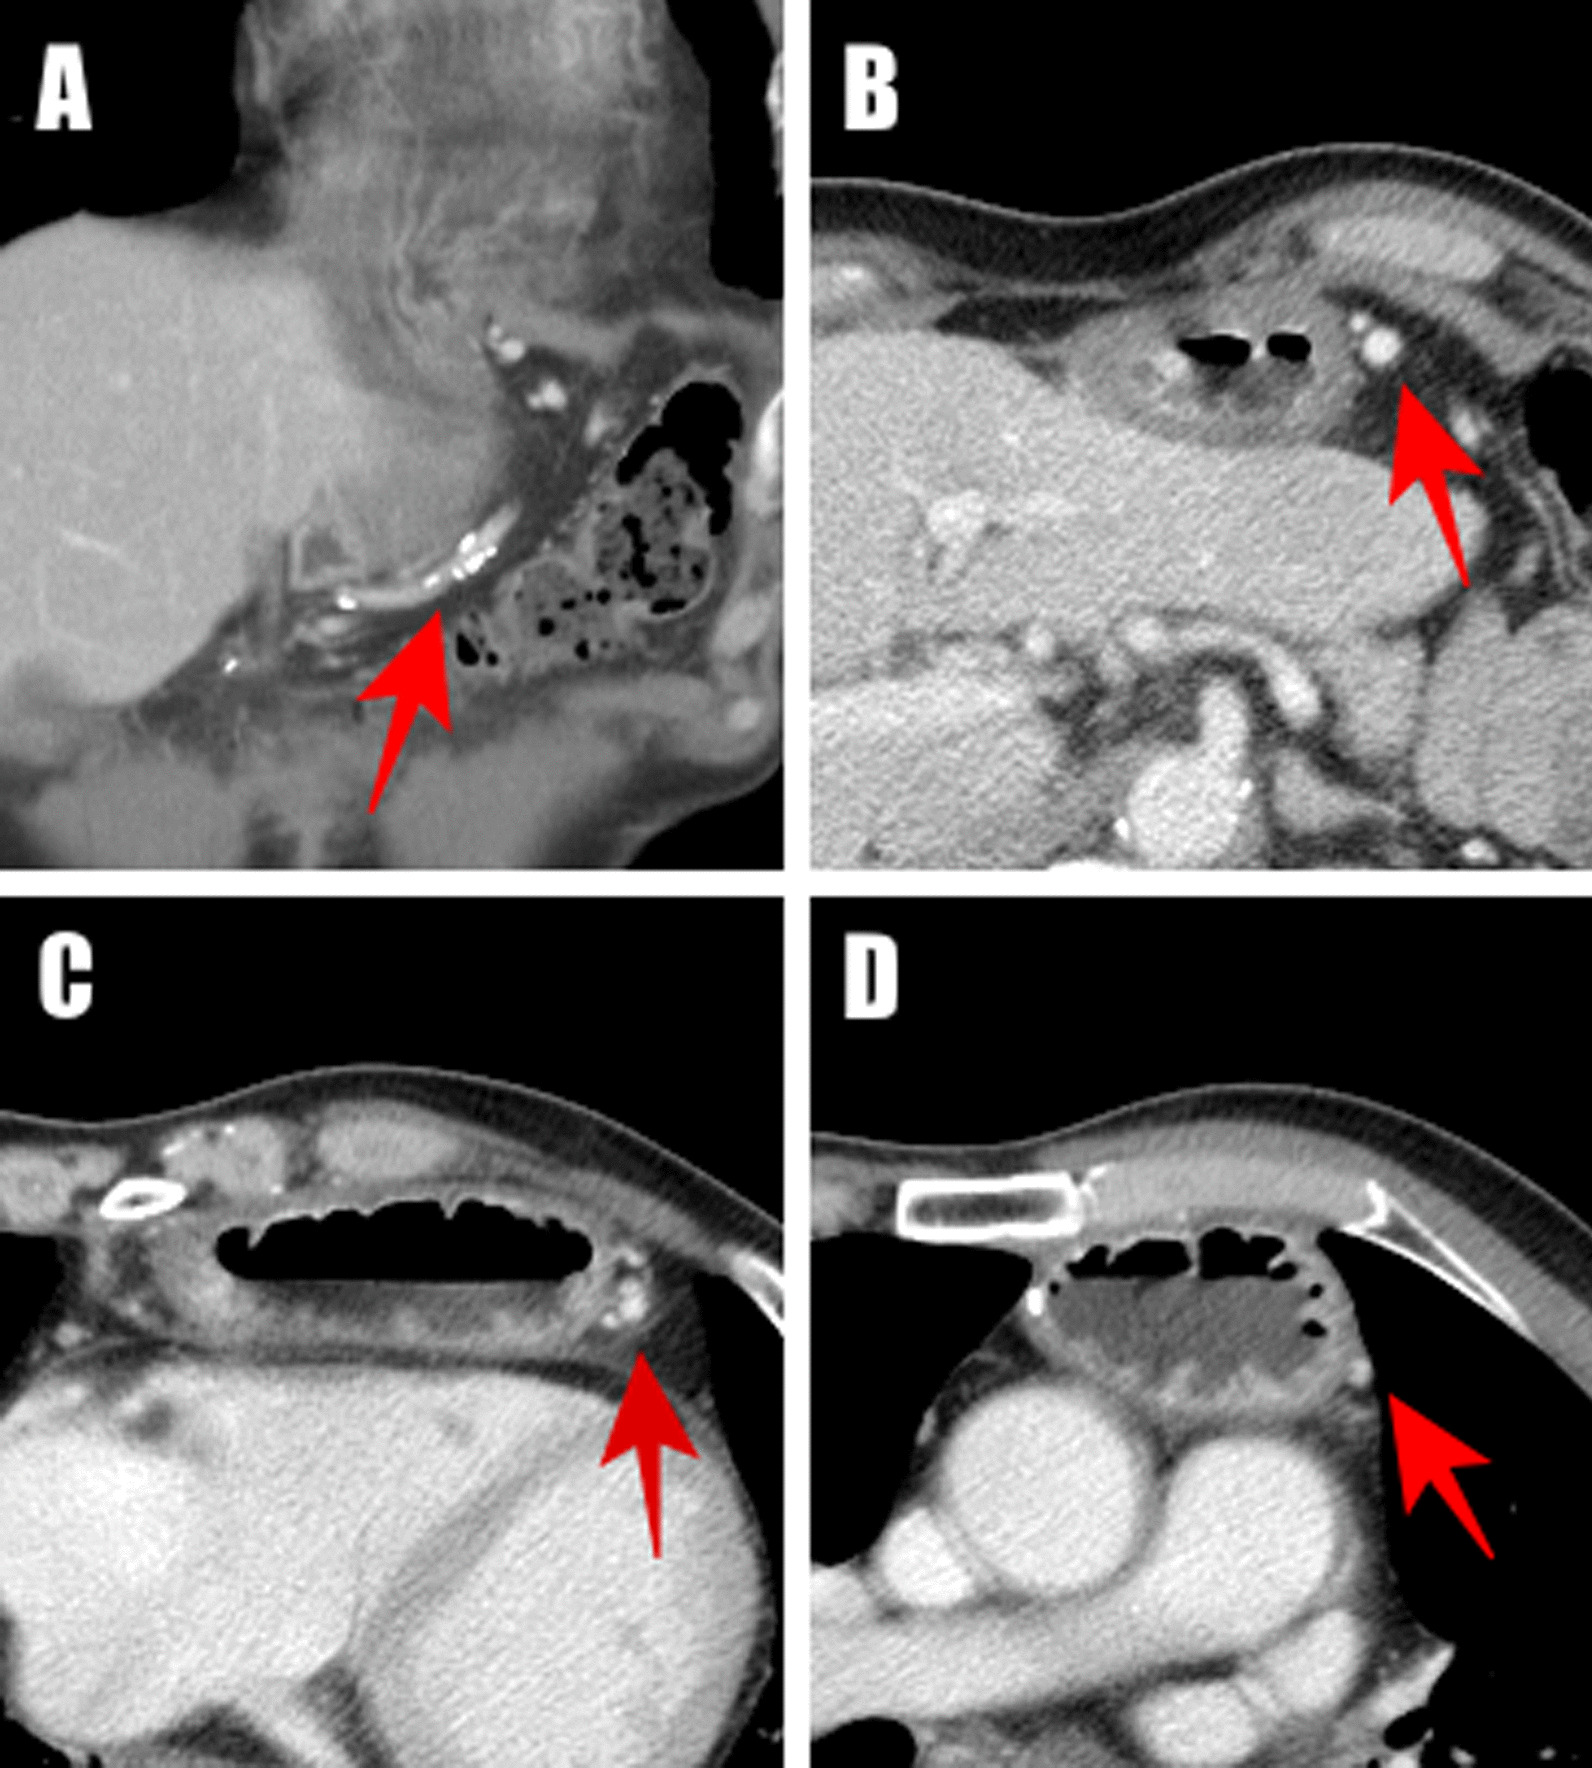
Postoperative contrast computed tomography scan at postoperative day 10 revealed a patent right gastroepiploic vessel (red arrow), which could be identified from the proximal (A, B) to the distal edge of the gastric conduit (C, D)
